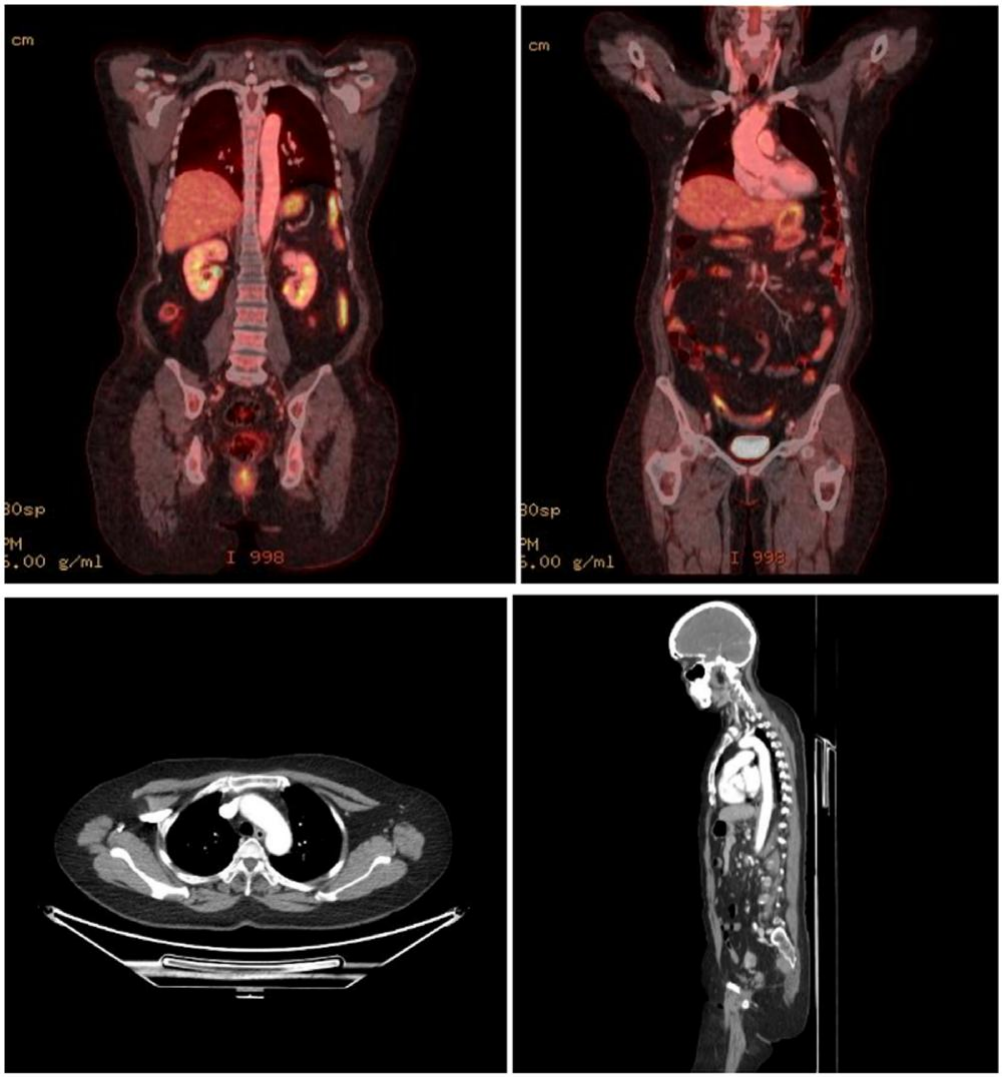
Post-symptom resolution positron emission tomography and computed tomography showing inflammation resolution.
